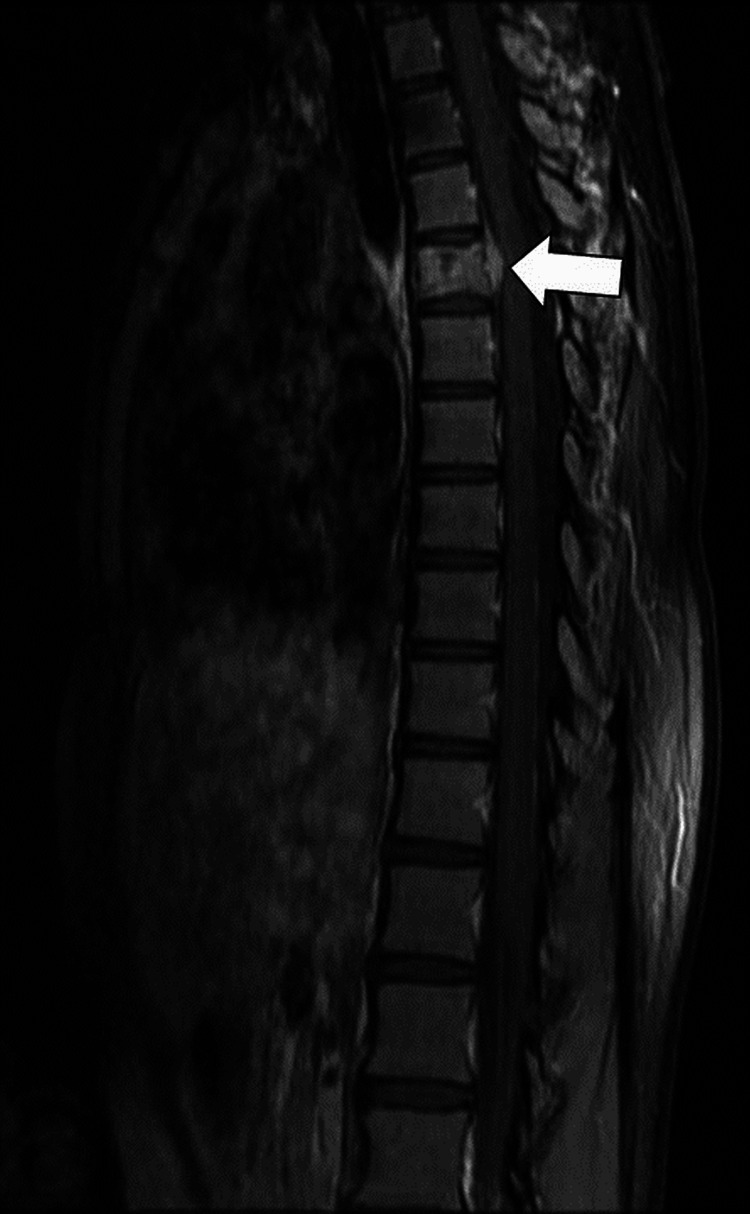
Saggital T2-weighted MRI shows a heterointense lesion (arrow) in the D5 vertebra with a significant reduction in vertebral body height consistent with vertebral metastasis.
